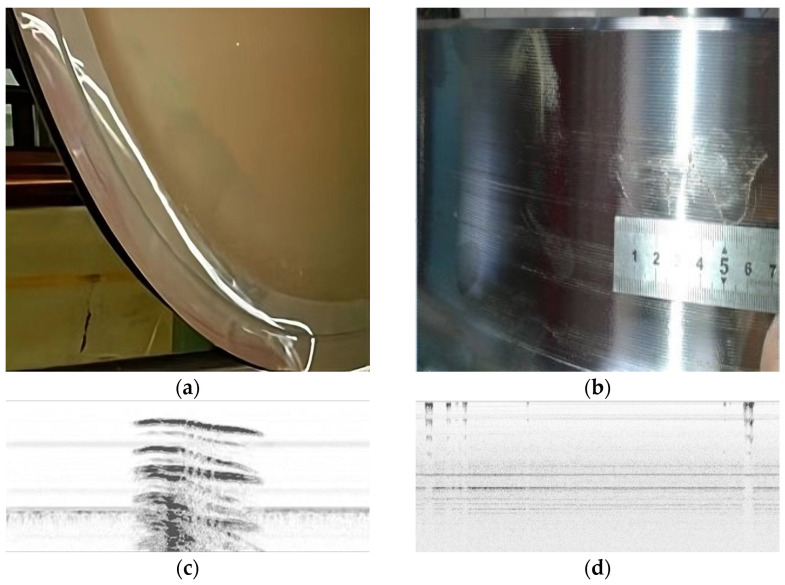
Typical defects: (a) wheel rim crack; (b) scratch; (c) ultrasonic b-scan defect image of rim crack; (d) surface and near-surface b-scan defect images.
